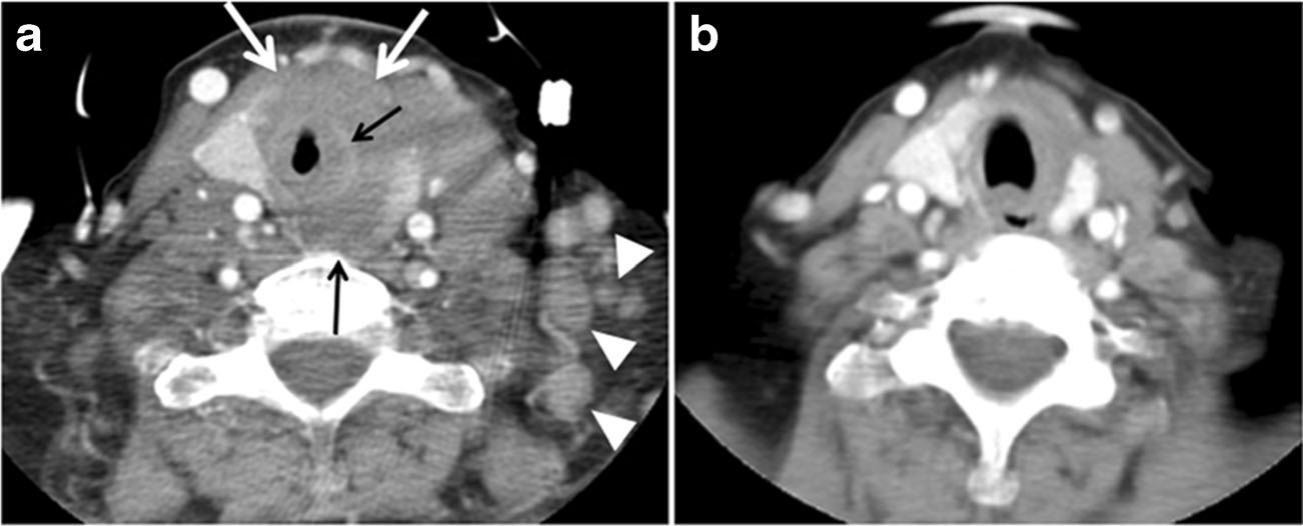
Thyroid non-Hodgkin’s large B-cell lymphoma in a 66-year-old female patient. a Axial enhanced neck CT scan demonstrates left thyroid lobe and isthmus homogeneously hypodense and minimally enhancing mass (*white arrows*). This lesion invades the prevertebral muscles (*black arrows*). Note the multiple enlarged level V lymph nodes (*white arrowheads*). b Post-treatment image shows significant reduction in size and mass effect of the left thyroid infiltrative mass, with almost complete resolution of the left cervical lymphadenopathy
